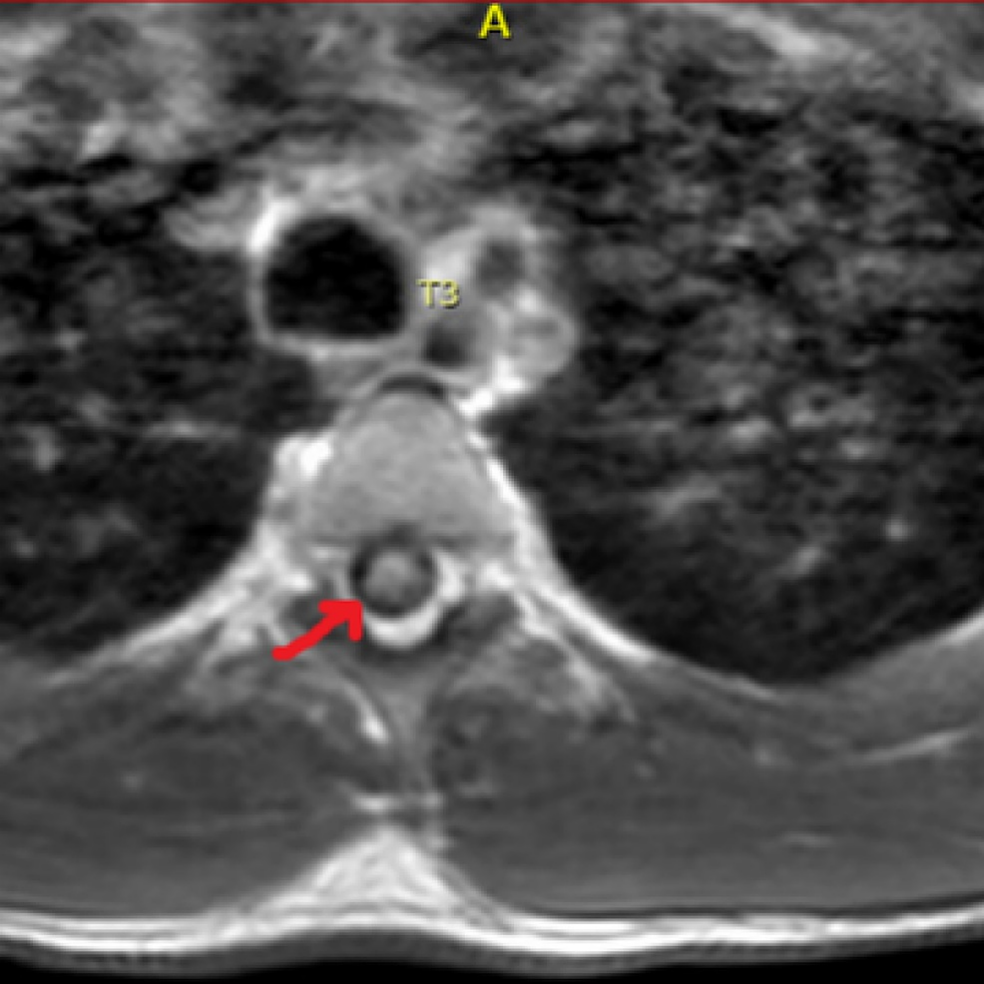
MRI of thoracic spine shows abnormal T2 signaling at the level of T3.
